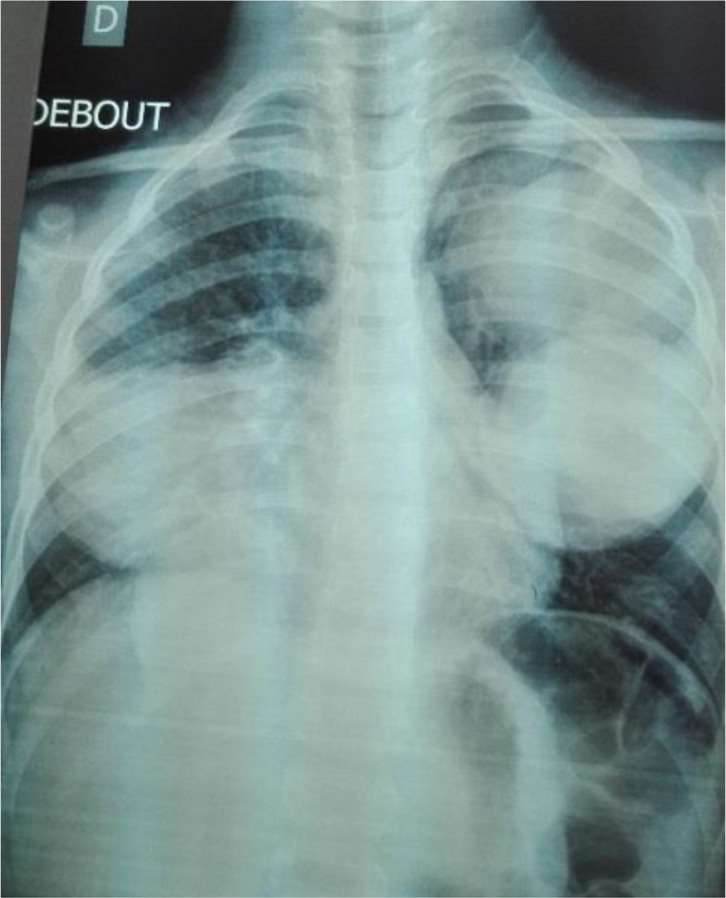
Radiography shows multiple hydatid cysts of the lungs, one in the right side and two in the left side
